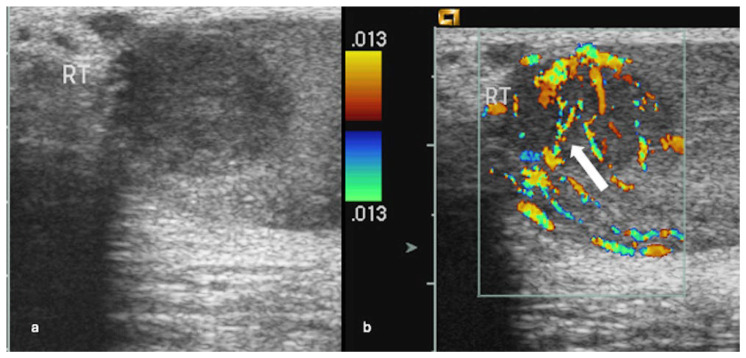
Disordered/haphazard intralesional vascular pattern in a mixed germ cell tumour. (a) Grey-scale ultrasound shows a well-circumscribed hypoechoic lesion in the right testis: (b) colour Doppler ultrasound demonstrates non-crossing disordered/haphazard intralesional vascularity (arrow).
